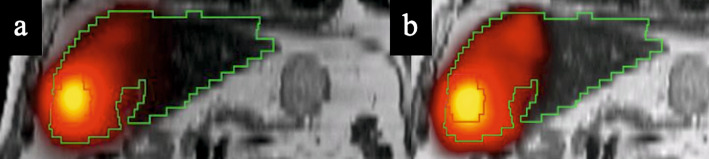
^166^Ho-PLLA SPECT/CT on MRI images (T2 sequence) for post-treatment dosimetry in a 84-years old man suffering from hepatocellular carcinoma through segment IVb, DEW method being applied on the left image (a) and TEW method on the right (b), tumor in red
